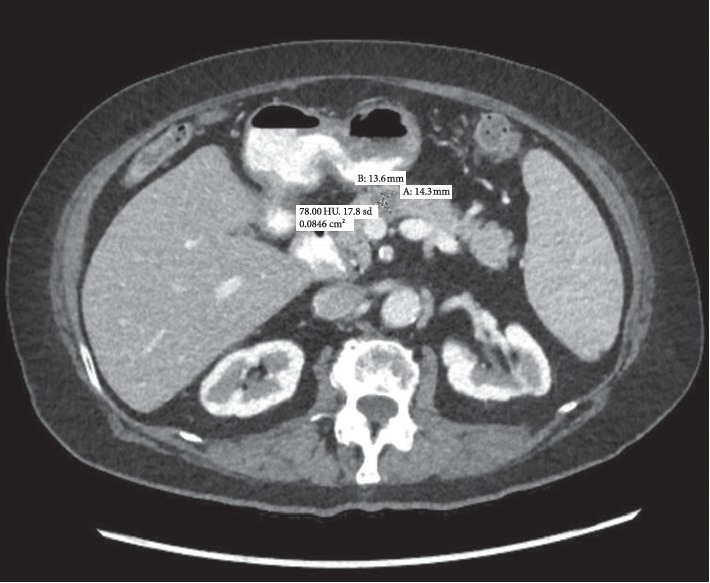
CT  abdo/pelvis: hypodense lesions within the pancreatic body and medial aspect of the pancreatic head which are concerning for a neoplastic process.
